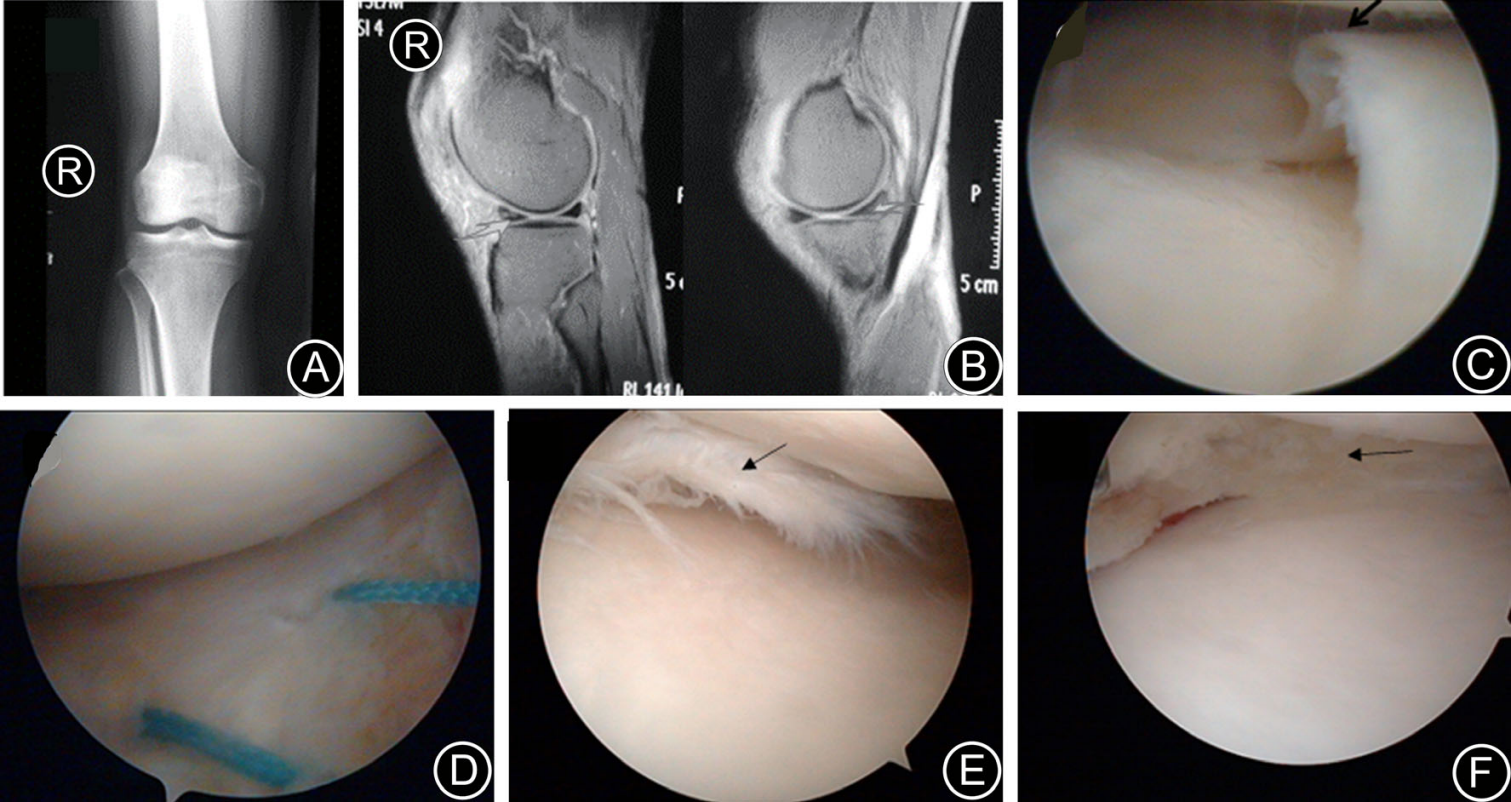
A 47‐year‐old male patient with combined injury of posterior horn medial meniscus and anterior horn lateral meniscus in the right knee. (A) Anteroposterior X‐ray film before operation; (B) Sagittal MRI before operation; black arrow indicates longitudinal tears of anterior horn lateral meniscus and horizontal tears of posterior horn medial meniscus; (C) Longitudinal tears of anterior horn lateral meniscus under arthroscope (arrow); (D) The anterior horn lateral meniscus after suturing; (E) Horizontal tears of posterior horn medial meniscus under arthroscope (arrow); (F) The posterior horn medial meniscus after menisectomy.
